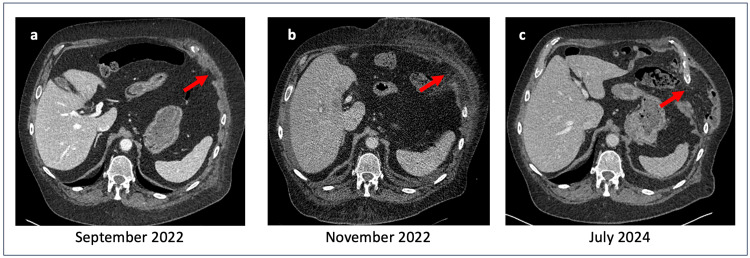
Sagittal view of thoracoabdominal CT scan showing the evolution of the left transdiaphragmatic intercostal hernia over time.
